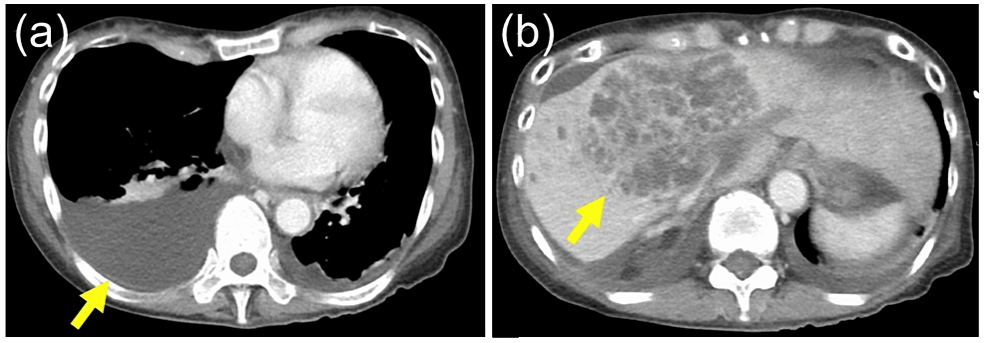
Contrast-enhanced computed tomography images at the time of transfer to our hospital Contrast-enhanced computed tomography at the time of transfer to our hospital revealed pleural effusion (a), an abscess of 10 cm in diameter in the liver anterior segment (b).
